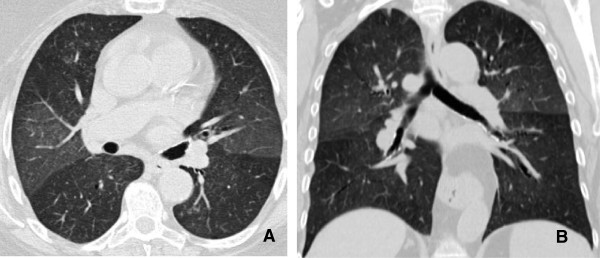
Interstitial pneumonitis. A and B: Axial and Coronal CT scan of the chest demonstrates diffuse areas of ground glass opacity predominantly involving the upper lobes associated with centrilobular nodules. There has been sparing of the lower lobes consistent with Rituximab induced interstitial pneumonitis.
